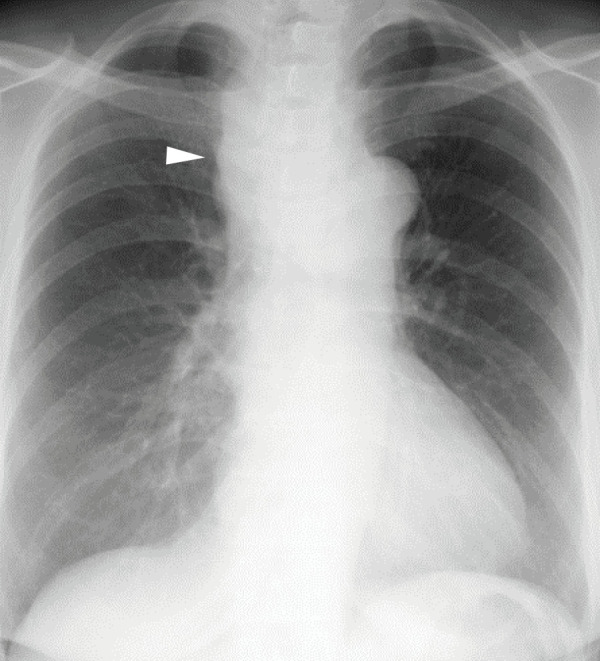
A chest radiograph showing a widened mediastinum (arrowhead).
